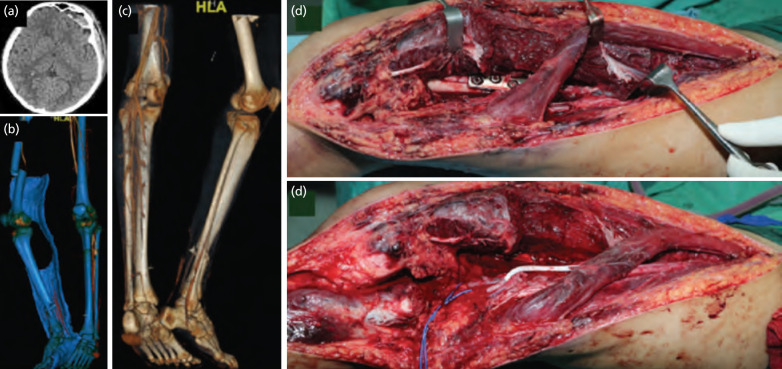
Case 2 – A 46-year-old man involved in road traffic accident whereby the thigh was rolled by other motorbikes. Trauma clearance revealed frontal contusion and multiple ribs fractures. (a) There is significant soft tissue contusion with fracture over midshaft femur and distal tibia. (b and c) CT angiogram showed complete filling defect over the fracture site with no distal run-off and collateral. (d and e) The circulation was established by PTFE graft 17 hour after injury however he developed renal impairments secondary to myoglobulinemia with high creatine kinase of more than 20,000 U/L. Secondary amputation was done at day 2 to minimise the systemic effect of reperfusion injury.
